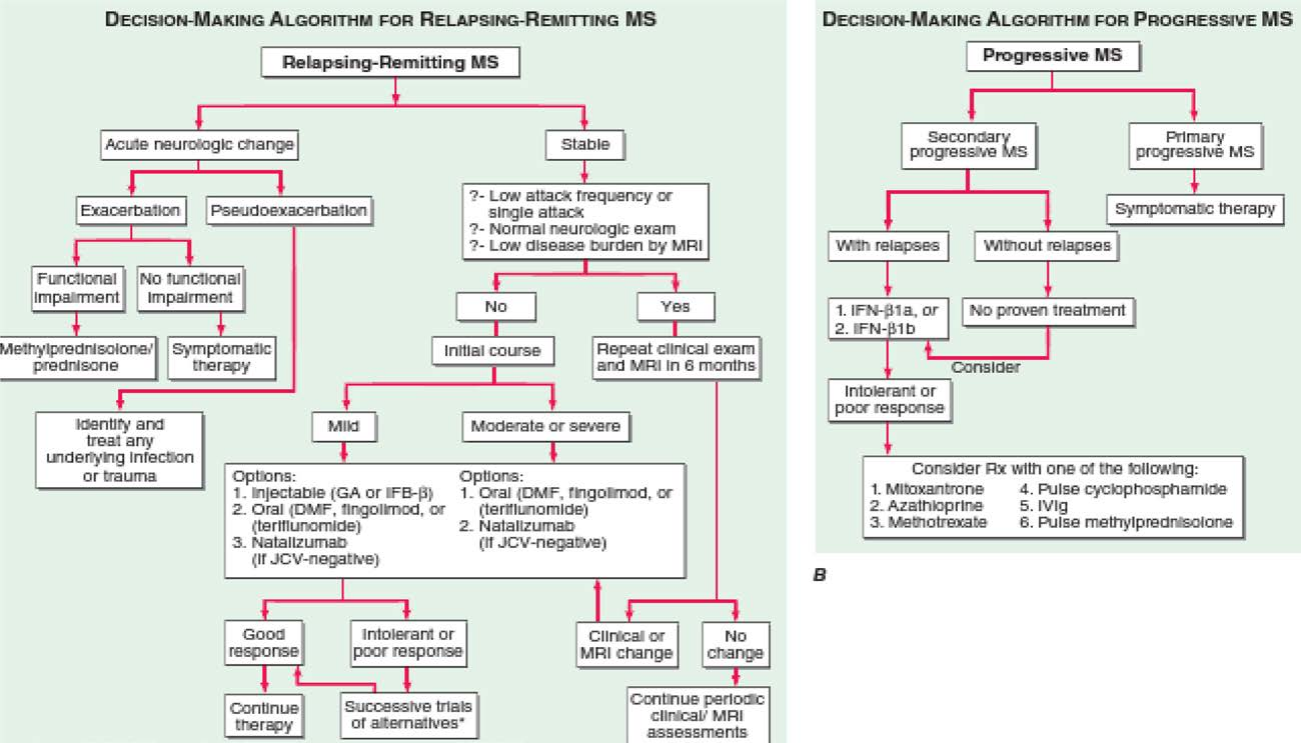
Multiple sclerosis treatment selection.^[36]^ It is possible to conduct studies of various interferon (IFN-) preparations, particularly moving from an Avonex-style once-weekly dose schedule to one that involves more frequent dosing (e.g., Rebif, Betaseron/Extavia). Additionally, natalizumab usage is an option for people who have the JC virus. The MRI, or magnetic resonance imaging. MRI = magnetic resonance imaging.
